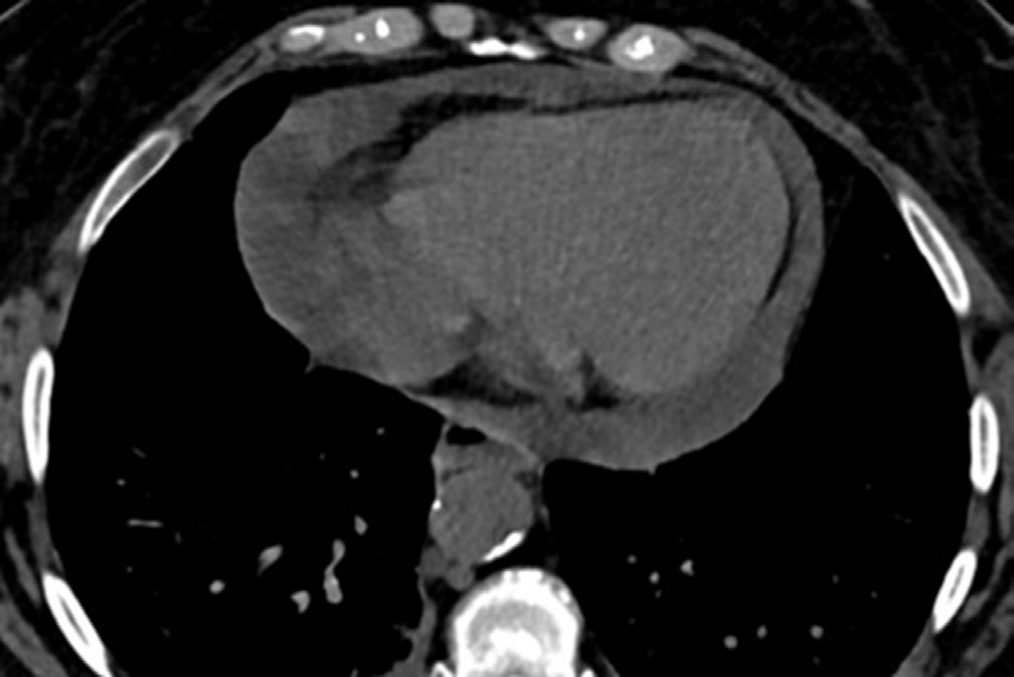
A 53-year-old female COVID-19 patient (Omicron variant), presenting with dry cough, dyspnea and fever up to 39° for 3 days. Axial CT image showed large amount of pericardial effusion.
